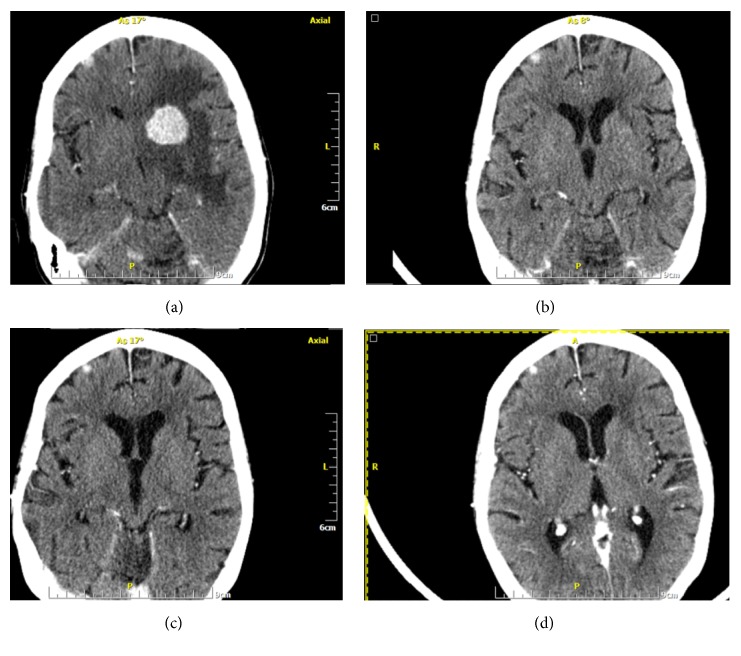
Brain computed tomography at baseline (a) and after 3 (b), 9 (c), and 12 (d) months of treatment with pertuzumab, trastuzumab, and taxane-based chemotherapy.
